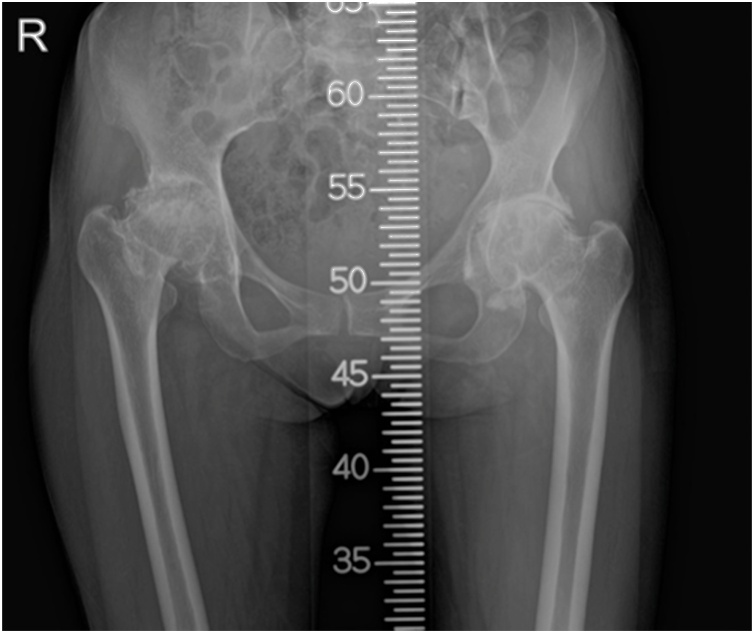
Radiography of both hips shows joint space loss, osteophyte formation, cyst formation and subchondral sclerosis.
